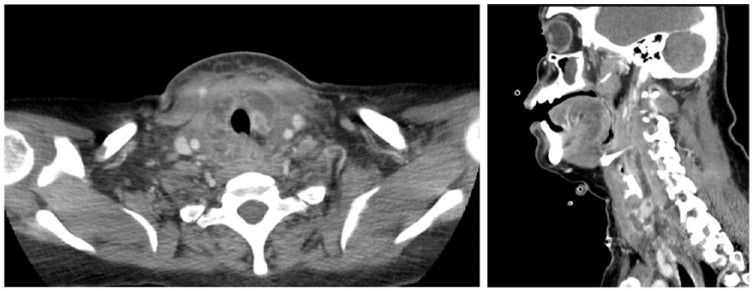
Computed tomography of the neck with contrast with extensive multilocular ring-enhancing lesions around the thyroid cartilage, extending superiorly into the retropharyngeal and prevertebral space at the C7-T1 level and extending posteriorly to the para-pharyngeal region.
